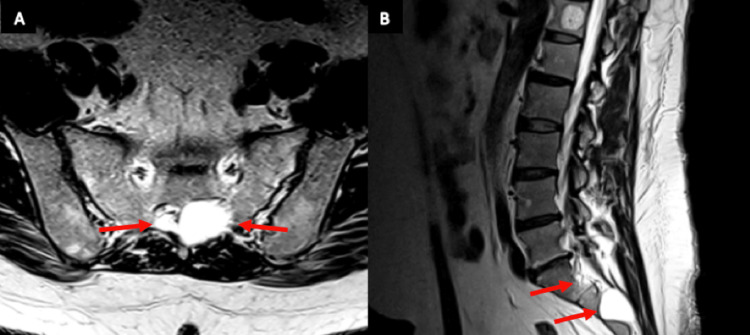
MRI views of Tarlov cysts affecting sacral nerve roots A: Axial MRI view. The red arrows identify multiple type II meningeal (Tarlov) cysts within the sacral foramina; B: Sagittal MRI view. The red arrows identify multiple type II meningeal (Tarlov) cysts at the level of S1-S2
